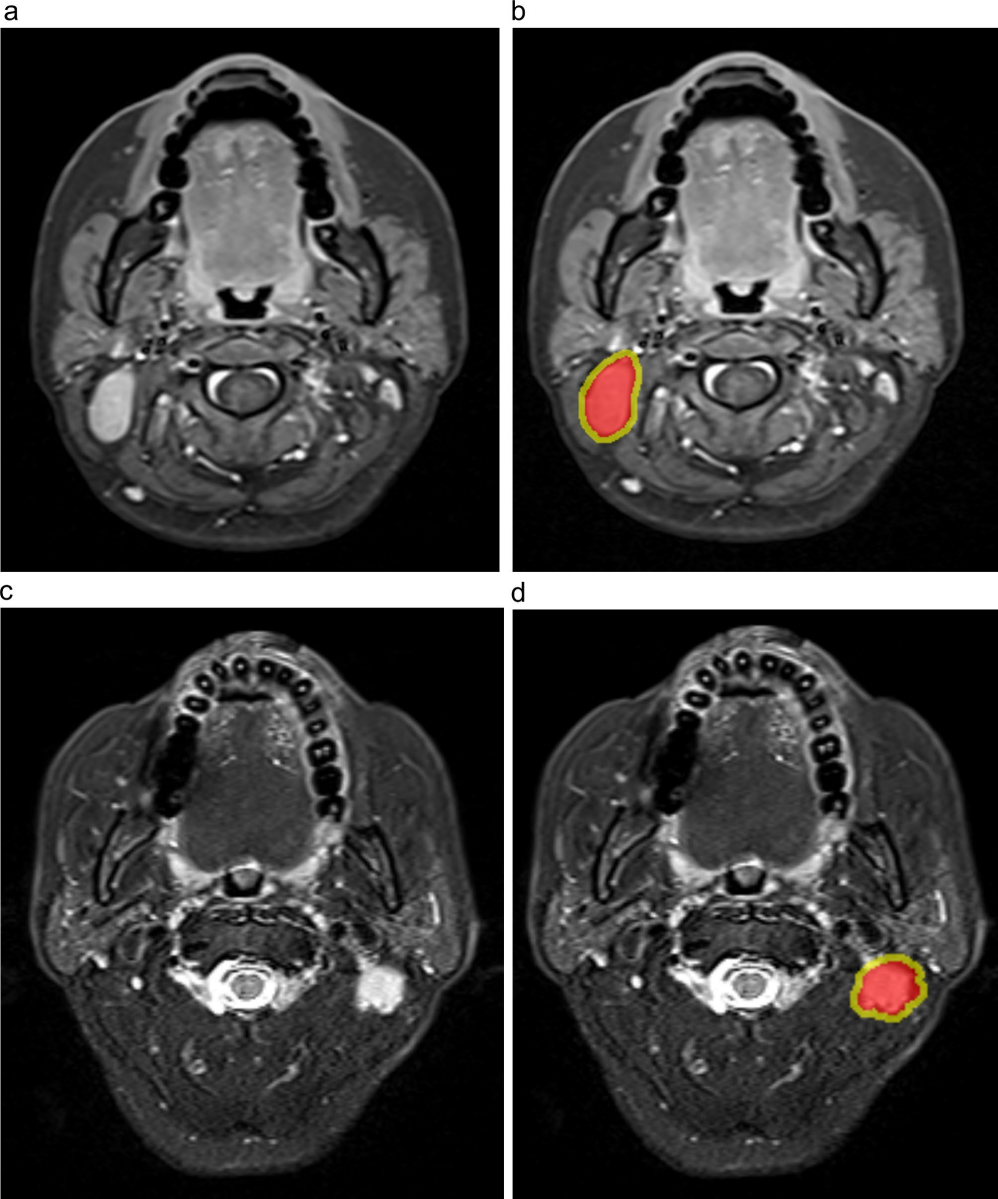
Representative slice of the MRI images and the corresponding Intra and Peri regions of interest (ROIs). The Intra ROI (red regions) drawn by a junior radiologist with the Peri ROI (yellow regions) generated by equidistant 3-dimensional dilation of the Intra regions with 2 mm. (a, b) Axial pretreatment CE-T1WI images. (c, d) Axial pretreatment T2WI images
